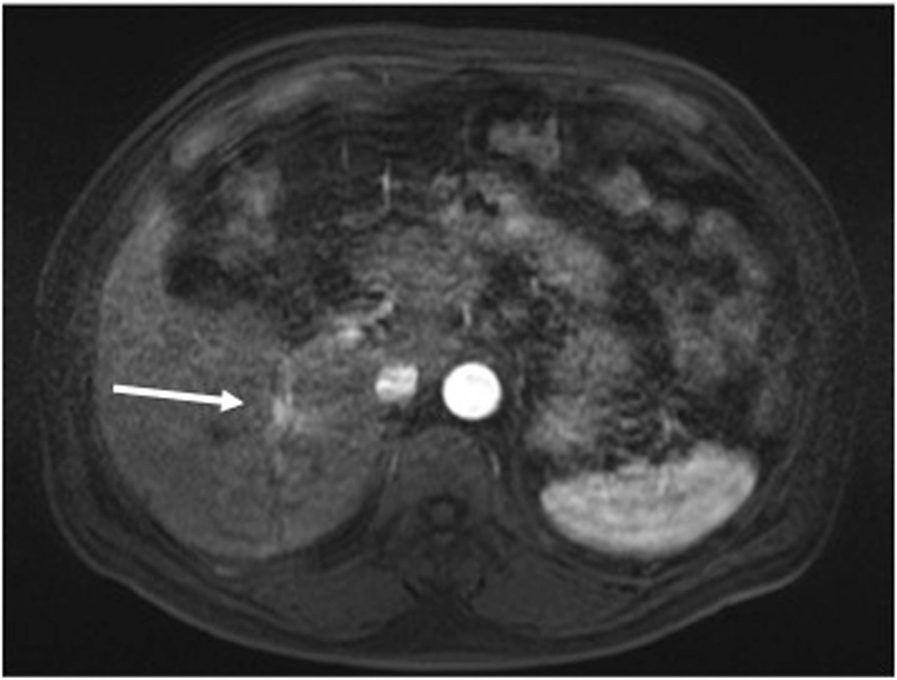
Enhanced magnetic resonance imaging after gadolinium injection. The tumor was hyper-intense on T1-weighted images (*arrow*) in the area adjacent to the main posterior biliary duct in the arterial phase but showed rapid washout in the late phase
